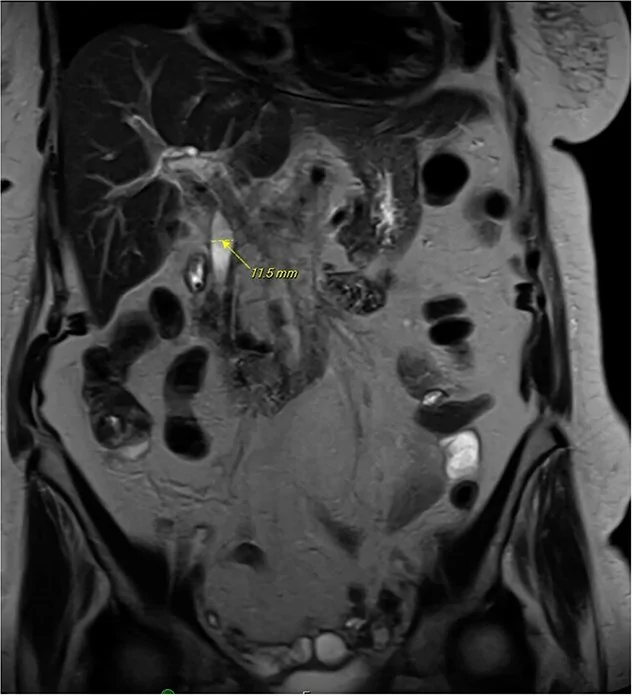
Coronal MRI showing a dilated CBD, highlighted by the arrow, without a discrete mass.
